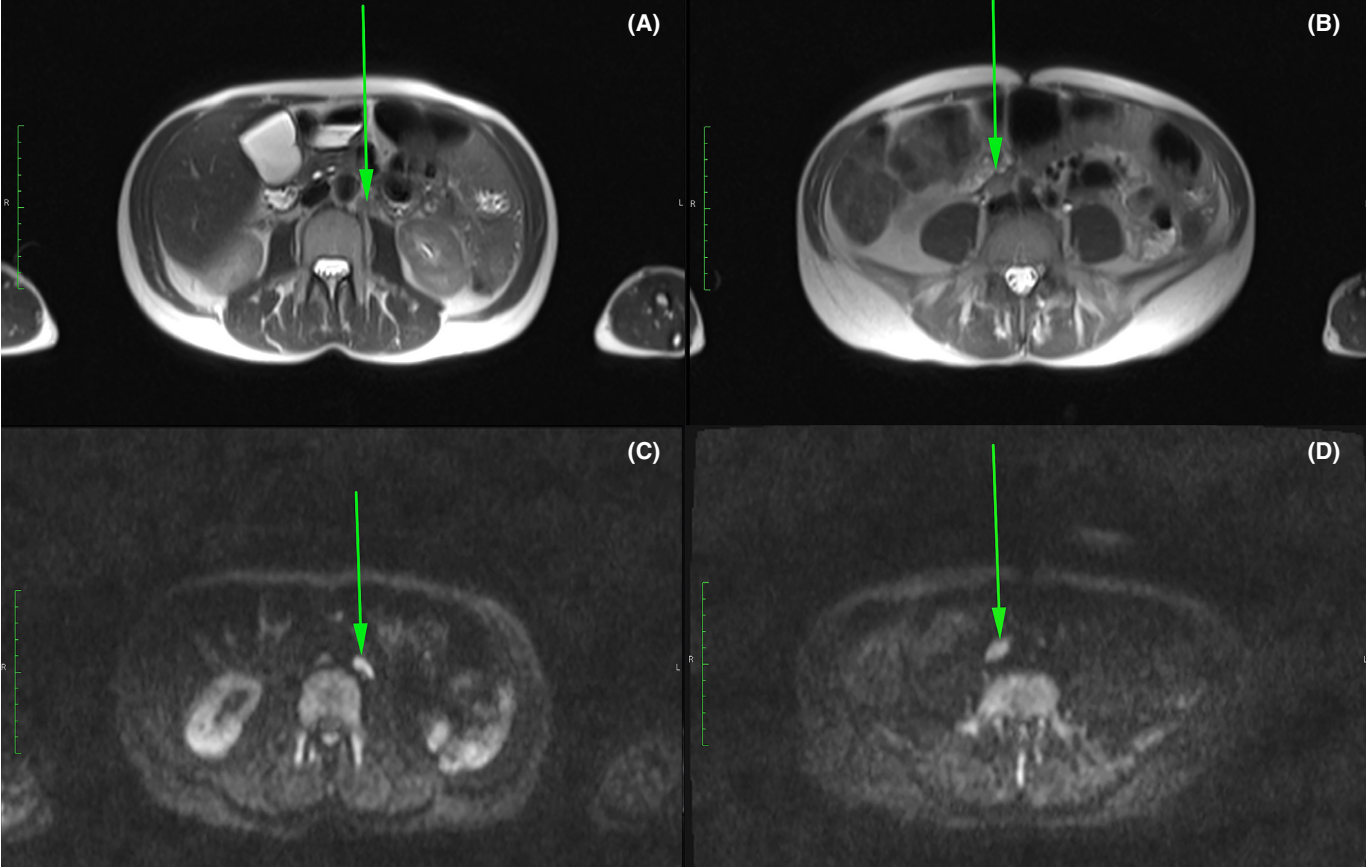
Magnetic Resonance Images (MRI) of the patient pre‐treatment, showing the metastatic lymph nodes in peritoneum and retroperitoneum (arrows) in t2 (A + B) and diffusion‐weighted (C + D) images.
